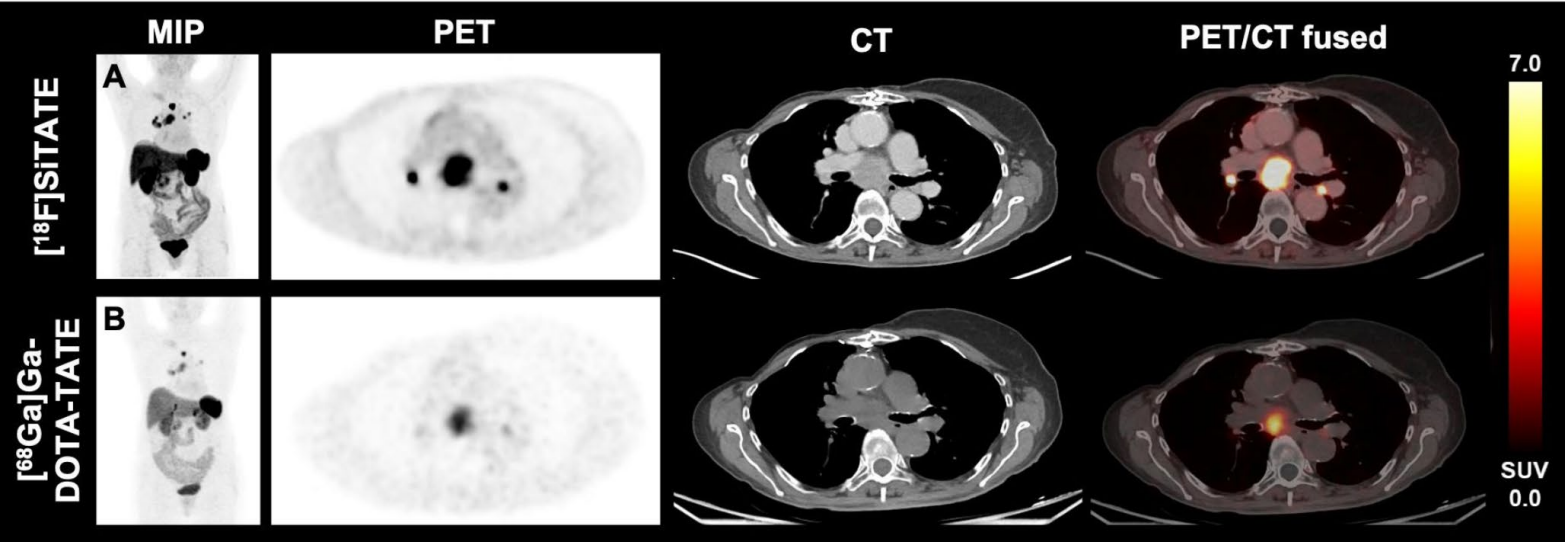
PET/CT imaging of a 75-year-old female patient diagnosed with FTC using the tracers [^18^F]SiTATE (A) and [^68^Ga]Ga-DOTA-TATE (B). The maximum intensity projection (MIP) and selected transversal PET and CT levels of the baseline and follow-up imaging are shown. The figure demonstrates mediastinal and hilar tumor manifestations. During the interval of 7 months the tumor volume increased by 29.2% and SUV_mean_ increased by 60.0%, suspicious of tumor progression
